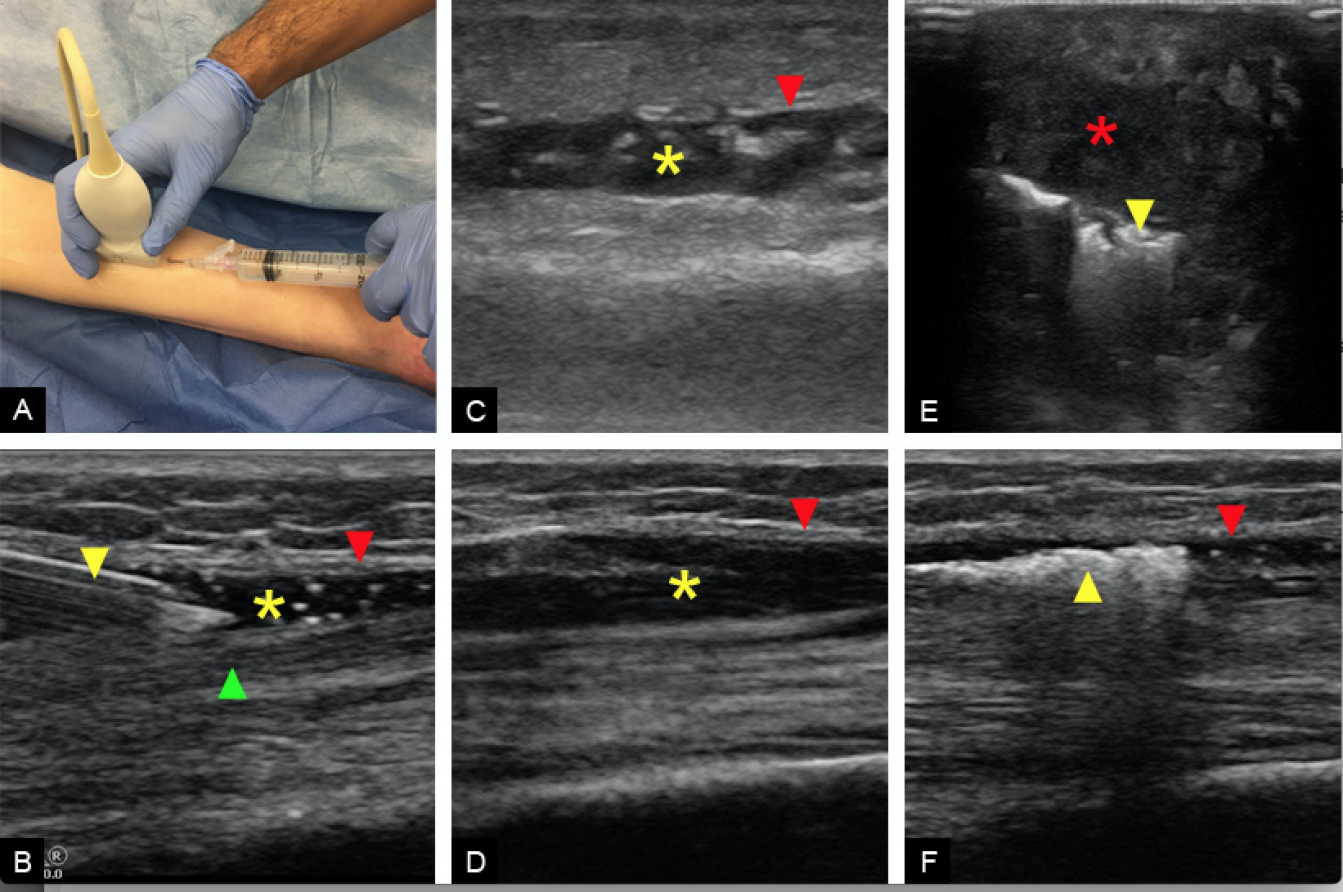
Preparation and Results A) Setup of the cadaver model showing ultrasound guided needle entry into the desired fascial plane. B) Sagittal ultrasound of the anterior lower extremity of the cadaver model showing needle guidance into the crural fascia; 18 gauge needle (yellow arrowhead), crural fascia (red arrowhead), tibialis anterior (green arrowhead), 1:1 mixture within fascia (yellow asterisk). C) Sagittal ultrasound of a real case of NF in the lower extremity showing fluid collection (yellow asterisk) below the crural fascia (red arrowhead). D) Cadaver model sagittal ultrasound of the lower extremity showing fluid collection (yellow asterisk) below the crural fascia (red arrowhead). E) Transverse ultrasound of a real case of NF in the foot showing hyperechoic gas collection (yellow arrowhead) within the plantar fascia deep to the subcutaneous tissue (red asterisk). F) Cadaver model sagittal ultrasound of the lower extremity showing hyperechoic gas collection (yellow arrowhead) within the crural fascia (red arrowhead).
